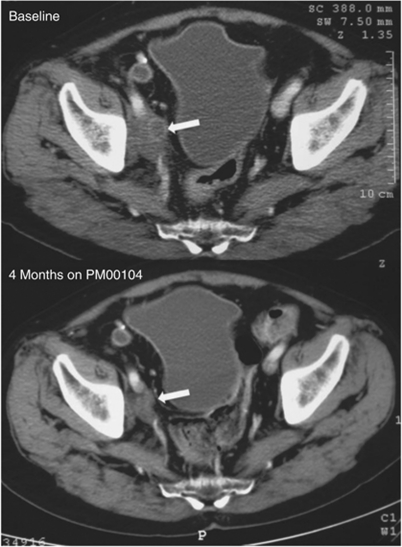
Pre- and post-treatment CT scans of the patient treated with four cycles of PM00104 3.5 mg m^−2^ 3-h 3-weekly and with partial response according to Response Evaluation Criteria in Solid Tumors (RECIST). These are CT scans from a 64-year-old male with metastatic urothelial cancer who had previously received cisplatin/gemcitabine, radiotherapy to the pelvis and carboplatin/paclitaxel. The maximum tumour shrinkage in the target lesion (right iliac lymph node) was 49.0% by RECIST measurements. However, disease progression was found in the brain after cycle 4, leading to drug discontinuation (time to progression of 4.2 months).
